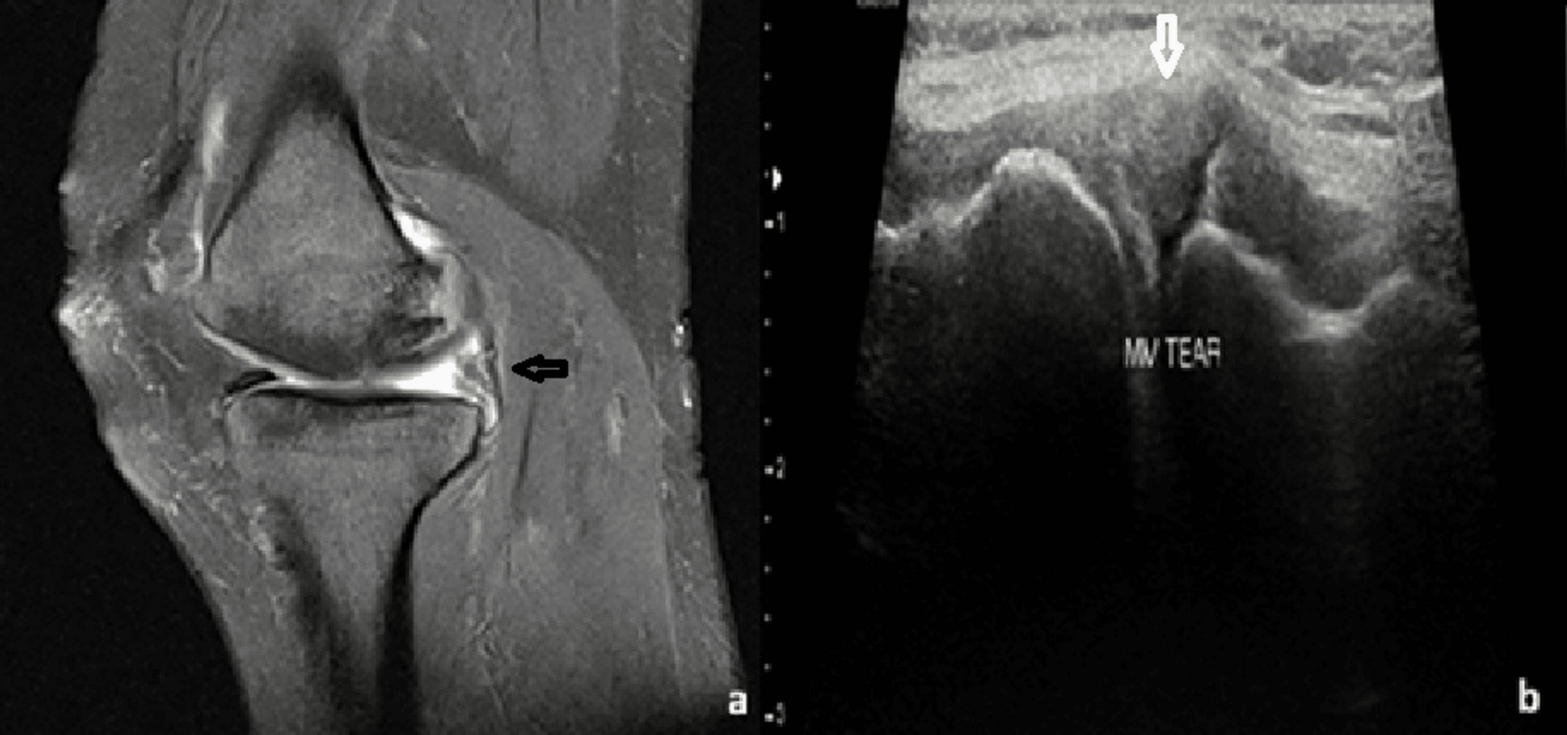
A 24-year-old male patient with a radial tear of the medial meniscus. (a) Sagittal PDFS MRI image demonstrating a radial tear involving the posterior horn of the medial meniscus, showing the characteristic “ghost sign.” (b) Corresponding USG image demonstrating a hypoechoic wedge-shaped area within the normal echogenic meniscus, consistent with a tear. PDFS: proton density fat suppression; USG: ultrasonography; MRI: magnetic resonance imaging
